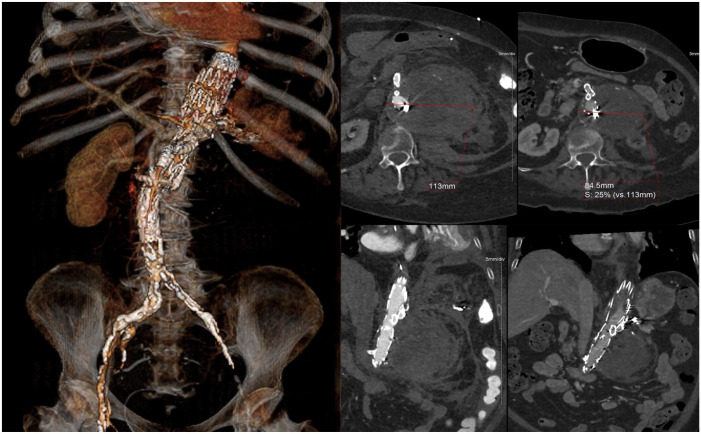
Three-dimensional rendering of the 6-month postoperative computed tomography scan and axial views showing shrinkage of the aneurysm sac. Red arrow indicates the access point to the common iliac artery from the common iliac vein.
